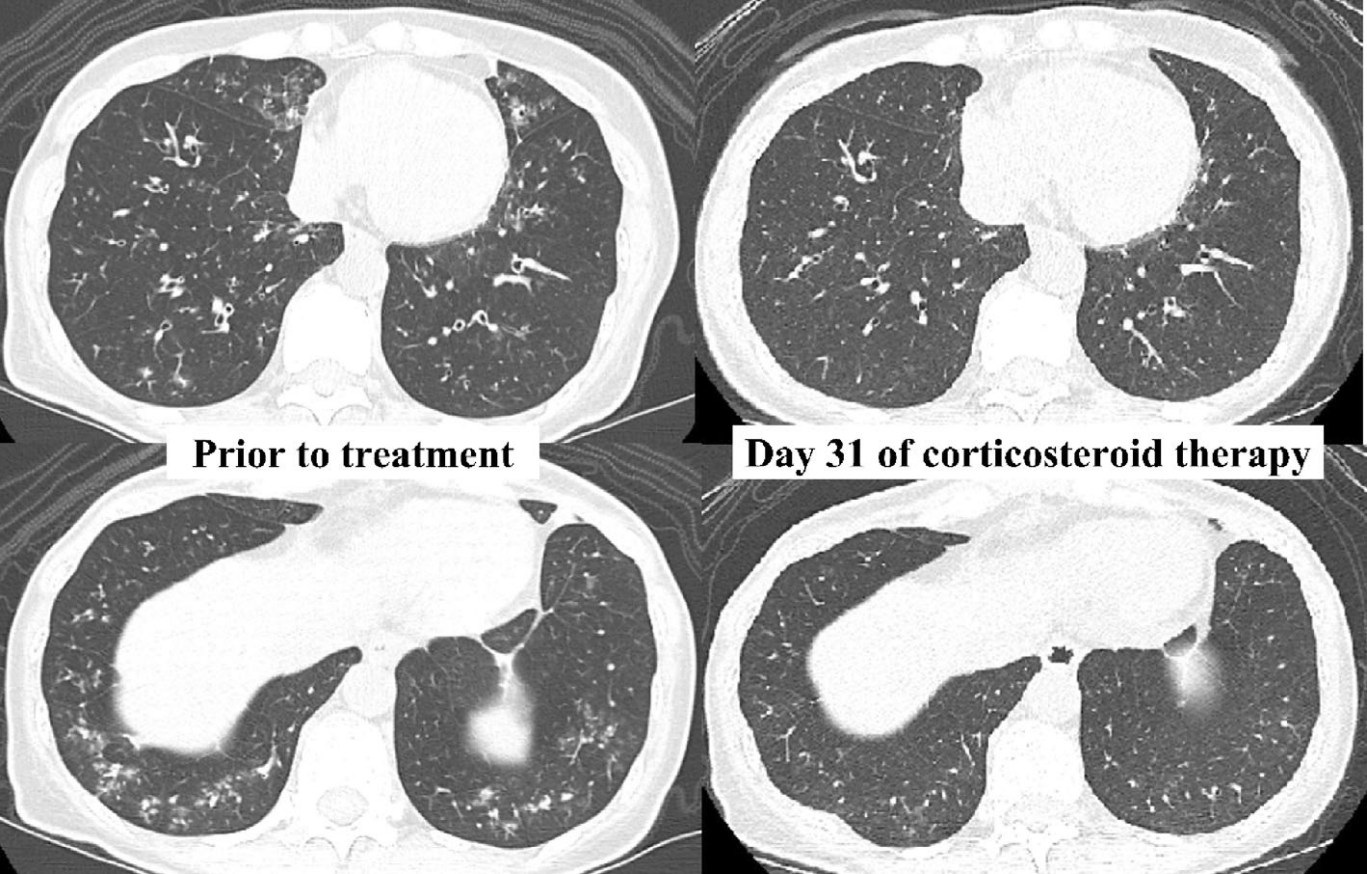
Chest computed tomography (CT) prior to (on the left side) and 31 days after (on the right side) the corticosteroid therapy revealing the improvement of eosinophilic bronchiolitis.
